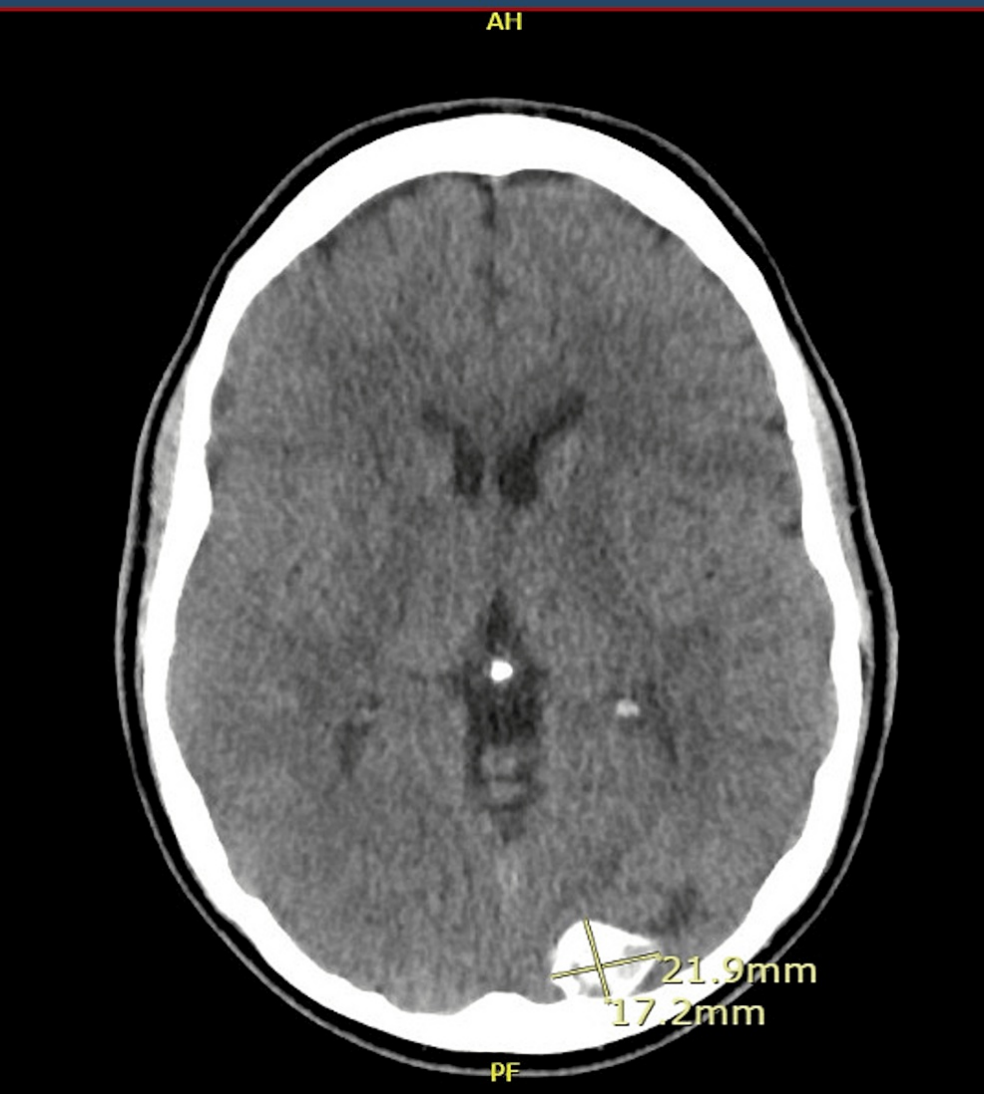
CT scan of the brain showing a meningioma
